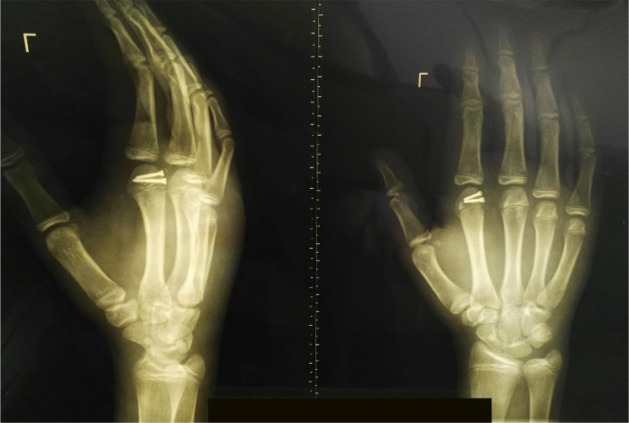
Two-year post-operation left hand oblique (right) and anteroposterior (left) radiographs
